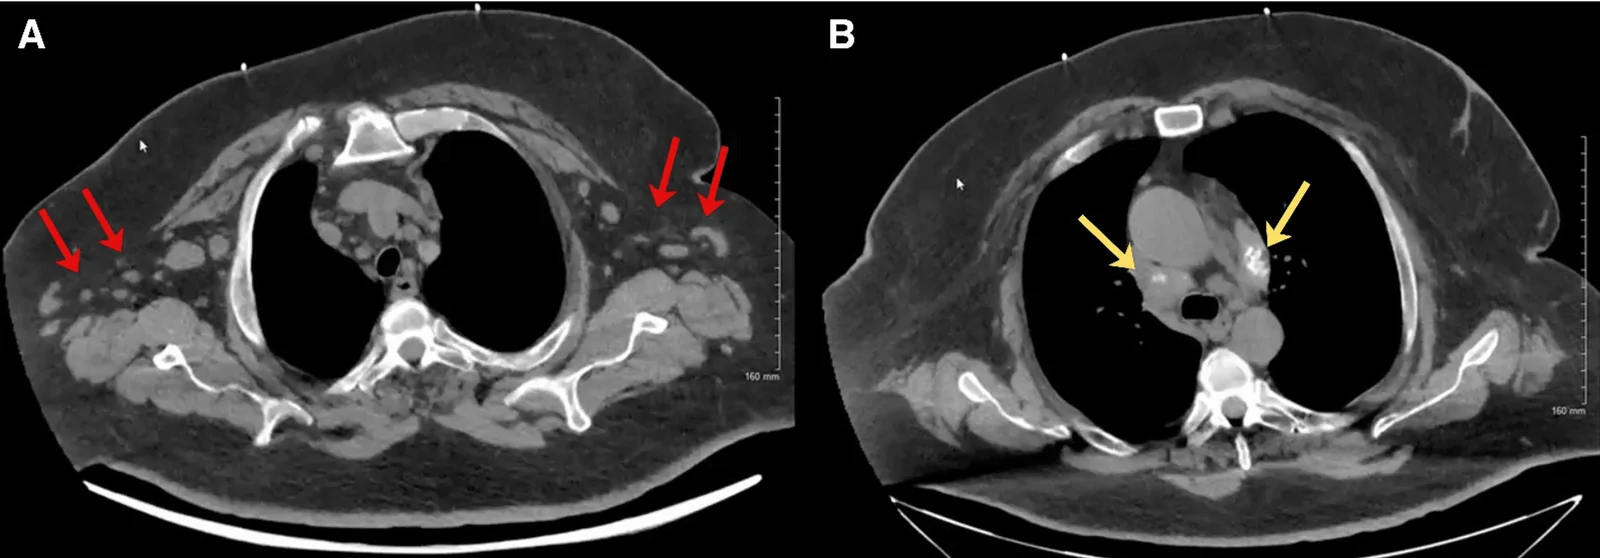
Computed tomography of the chest demonstrating bilateral axillary and mediastinal lymphadenopathy A: bilateral enlarged axillary lymph nodes (red arrows); B: enlarged calcified mediastinal and hilar lymph nodes (yellow arrows).
